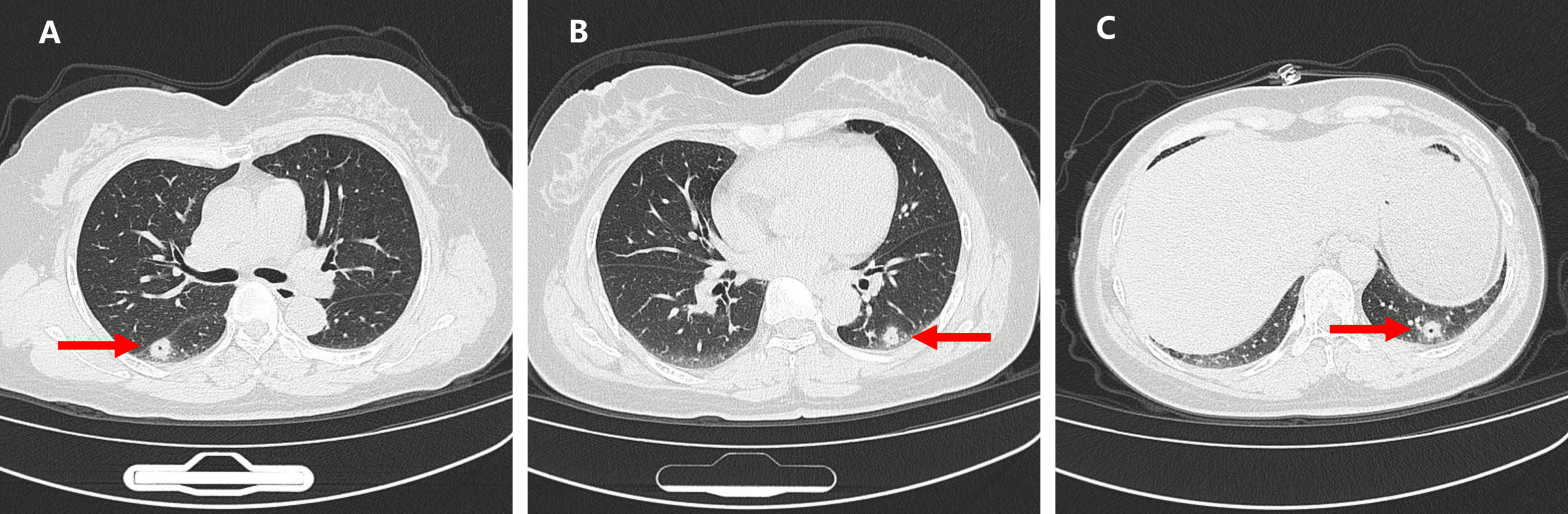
Chest CT on March 24, 2025, showing multiple subpleural thick‐walled cavities in both lungs (red arrows). The cavities demonstrated distinctive features: Round or oval consolidations with thick walls, small central cavities (approximately 1 mm in diameter) and characteristic subpleural distribution, predominantly located in the lower lobes.
